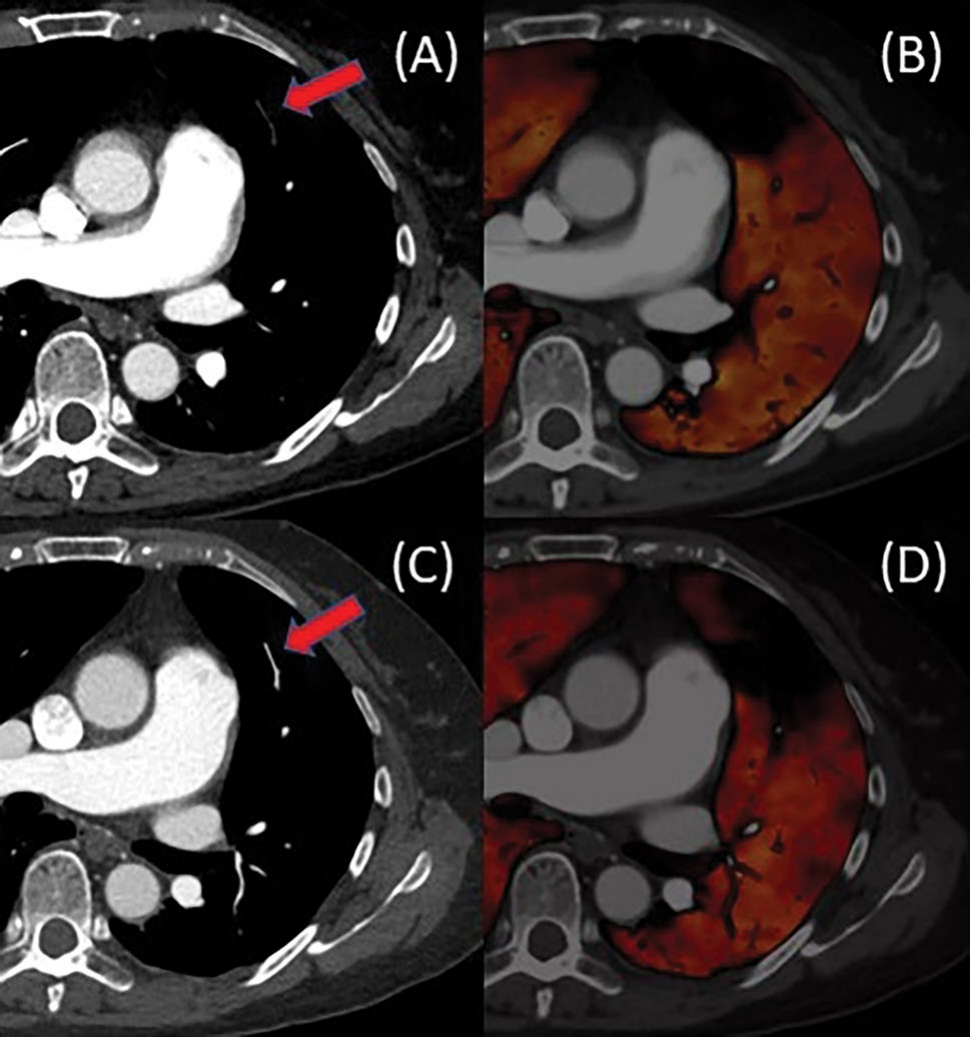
A 66-year-old woman with pulmonary embolism. (A) Pre-treatment computed tomography pulmonary angiography (CTPA) indicates peripheral occlusive thrombi of Lt. A4b. (B) Pre-treatment perfused blood volume shows perfusion decrease. (C) The thrombi disappeared on post-treatment CTPA. (D) Although the thrombi disappeared, the perfusion decrease remains on post-treatment lung perfusion blood volume.
